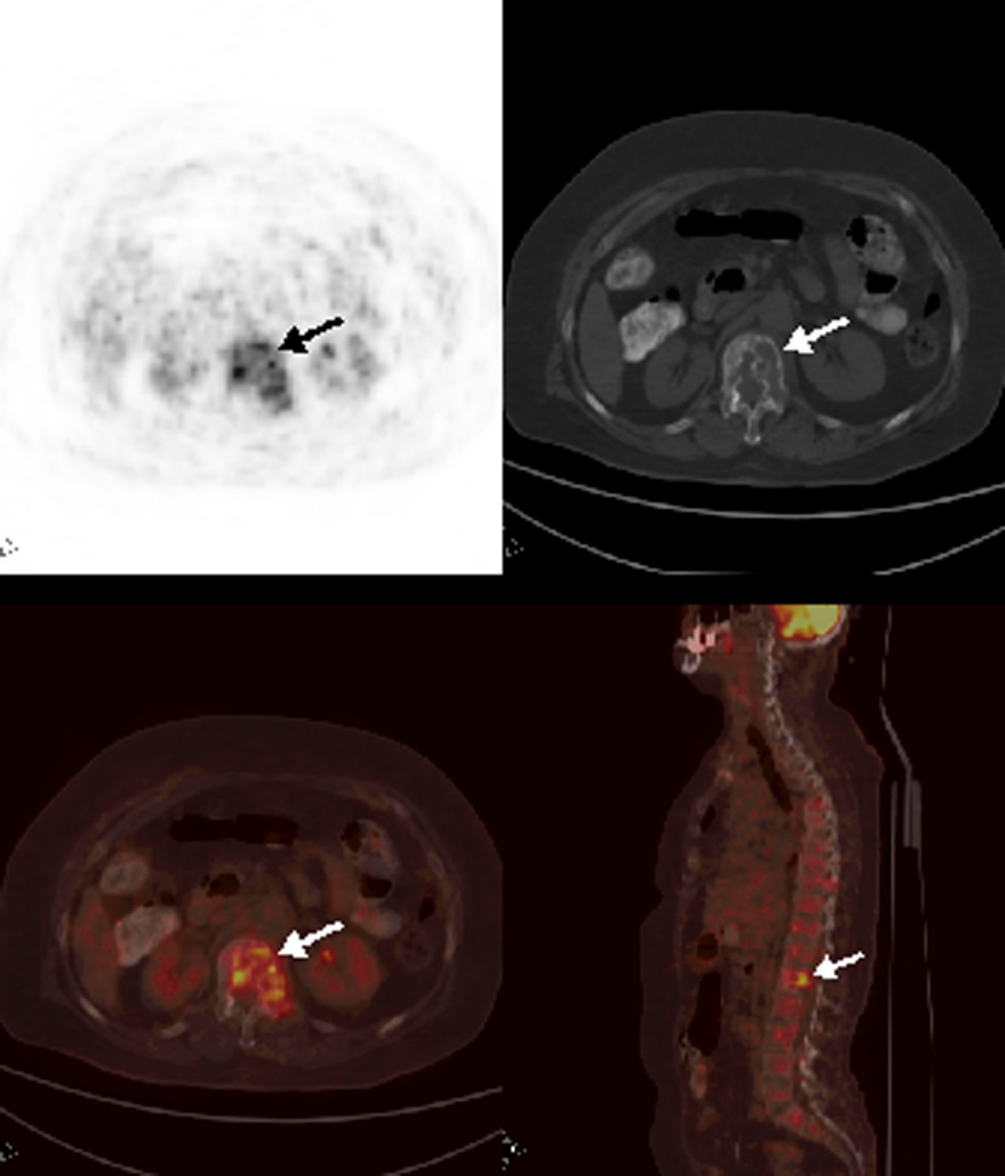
Axial and sagittal PET/CT images depicted multiple heterogeneously increased FDG uptake at vertebral column.
